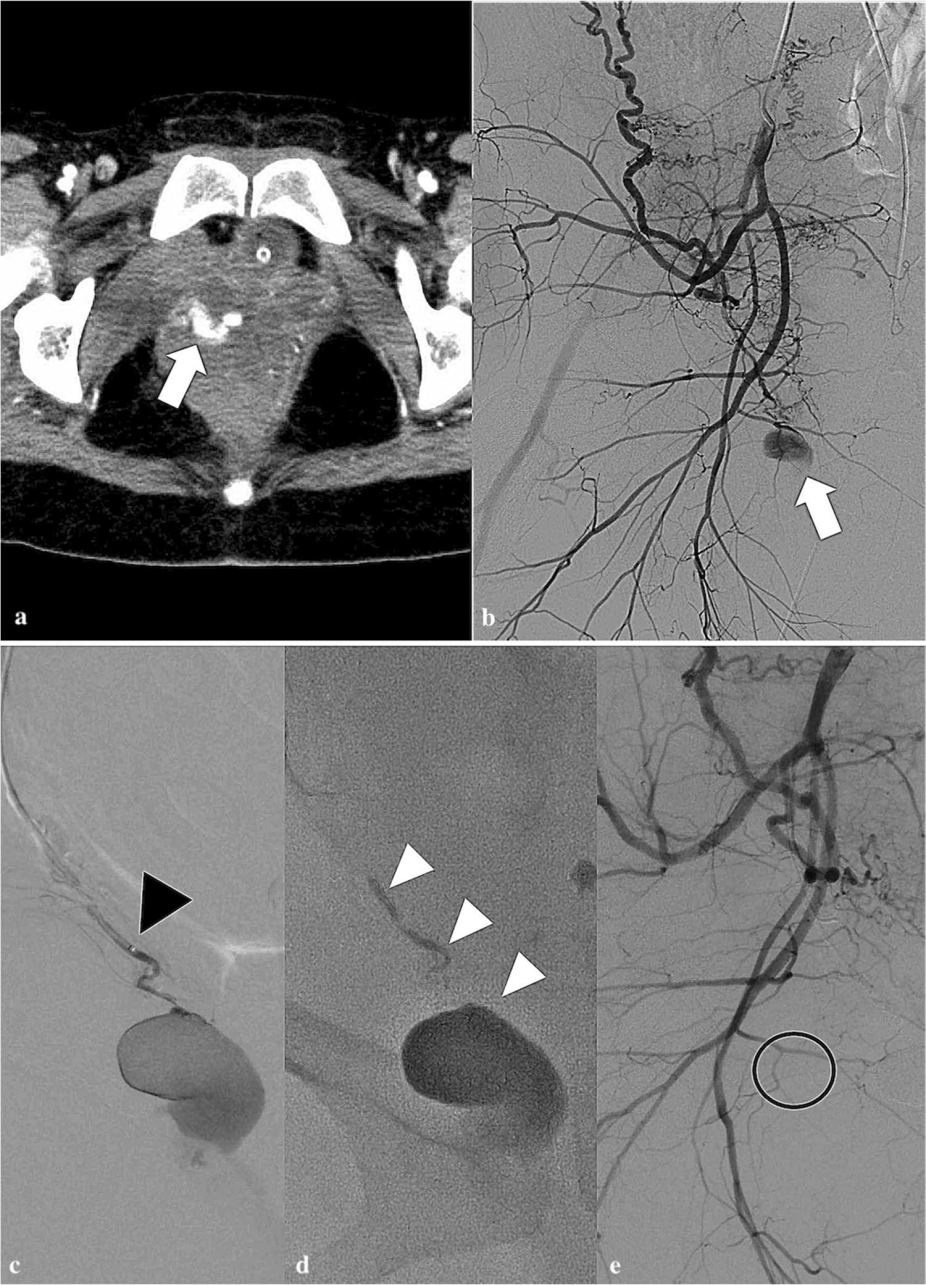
A typical case of superselective transcatheter arterial embolization. A 32-year-old woman with right vaginal hematoma presenting with postpartum hemorrhage after vaginal delivery. a Pre-procedural contrast-enhanced computed tomography shows extravasation of contrast medium in the right vaginal wall (white arrow). b Right internal iliac arteriography demonstrates a pseudoaneurysm from the right vaginal artery (white arrow). c Superselective angiography from the right vaginal artery; the black arrowhead points to the tip of the microcatheter. d The right vaginal artery was embolized with 33% NBCA-lipiodol mixture (white arrow heads). e The right internal iliac arteriography after embolization demonstrates disappearance of the pseudoaneurysm (black circle)
